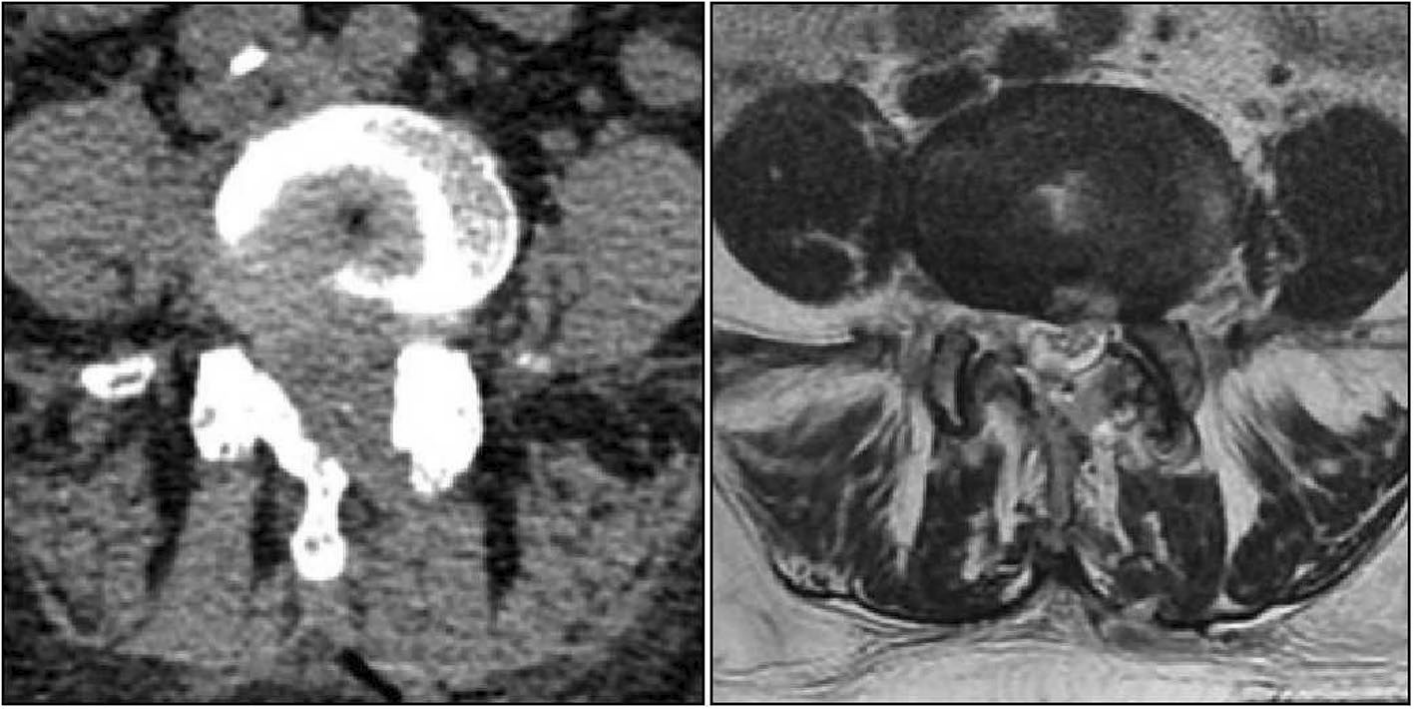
Postoperative follow-up MRI and CT scan demonstrated a significant increase in spinal canal volume when compared to the preoperative imaging.
